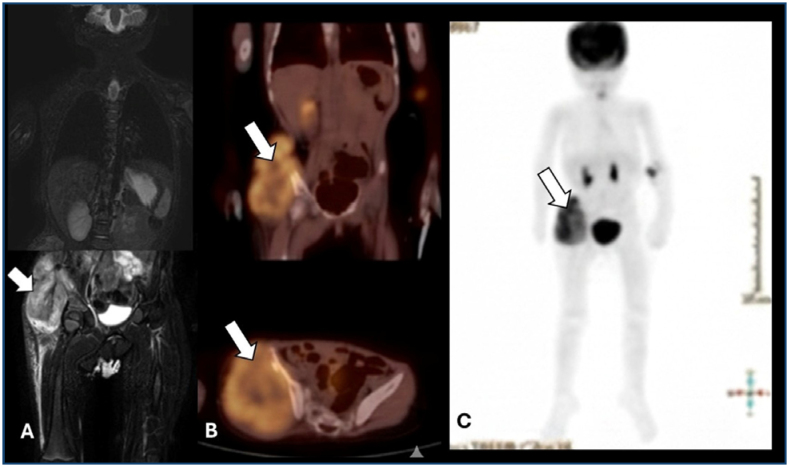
(A–C) 3-year-old girl infantile fibrosarcoma: coronal whole-body magnetic resonance imaging short tau inversion recovery image (A) demonstrates the right hemipelvic hyperintense solid mass (white arrow). Positron emission tomography-computed tomography fusion coronal and axial images show the right hemipelvic solid mass (white arrows) with an increased Fludeoxyglucose F 18 uptake (B). Positron emission tomography-computed tomography metabolic map image (C) shows Fludeoxyglucose F 18 uptake compared to surrounding tissues (white arrow).
